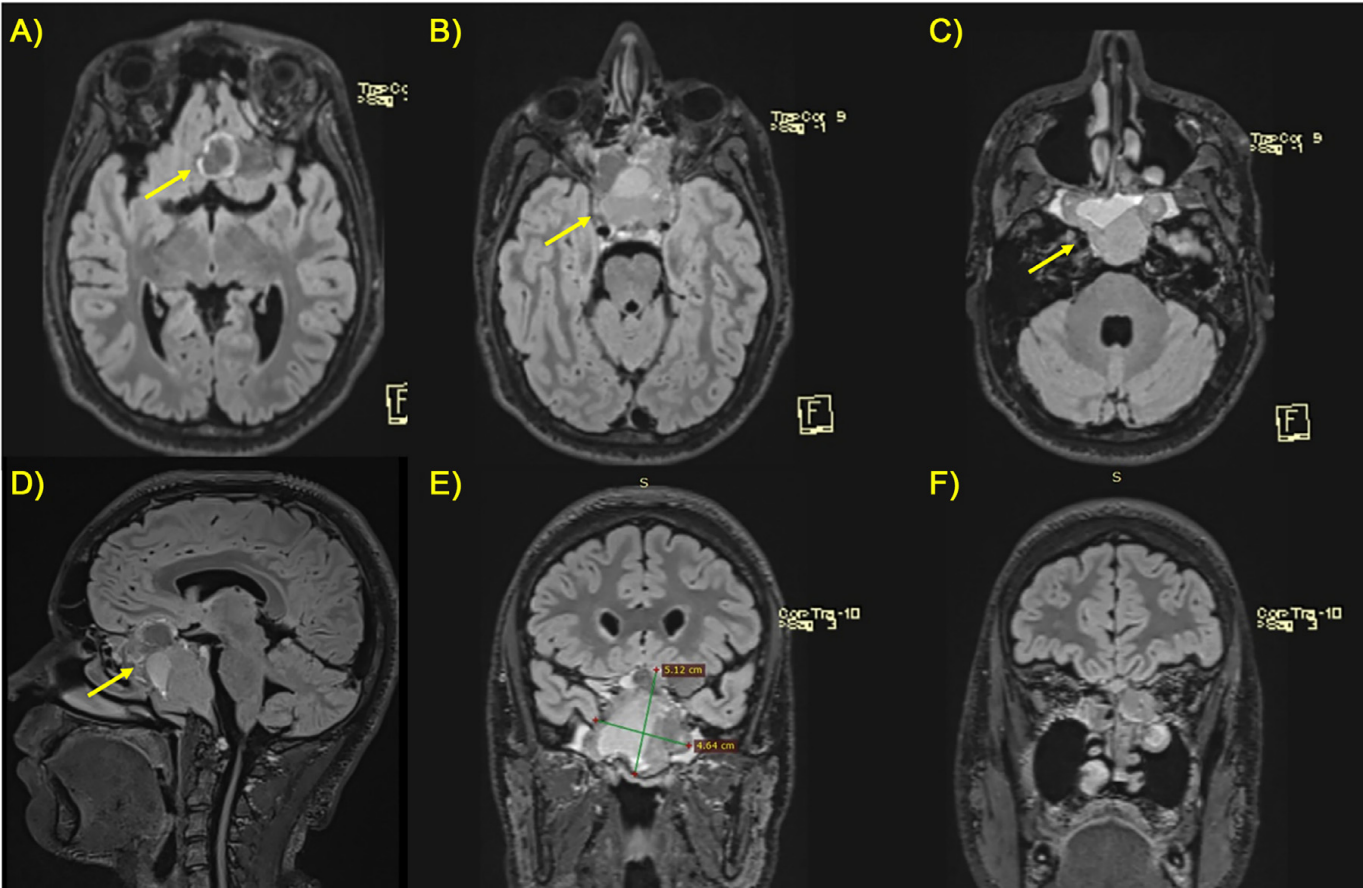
(A-F) FLAIR sequences on 3T MRI. Sequential axial (A-C), sagittal (D) and sequential coronal (E, F) planes showed a voluminous lobulated heterogeneous mass (yellow arrow) originating from the clivus, not recognizable, and extending in the adjacent structures. The mass presented mamelons/vegetations protruding into the nasal cavity, especially on the left side.
